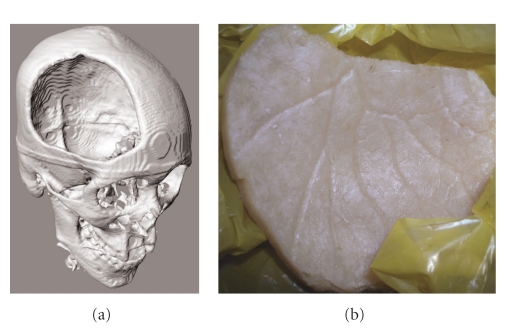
(a) Three-dimensional reconstruction of skull defect based on computed tomography (CT) scans of a patient who had a right frontoparietal craniectomy. (b) The arborizing course of the anterior branch of the middle meningeal artery can be observed on the internal aspect of the bone flap, providing a model for design of vascular channels.
